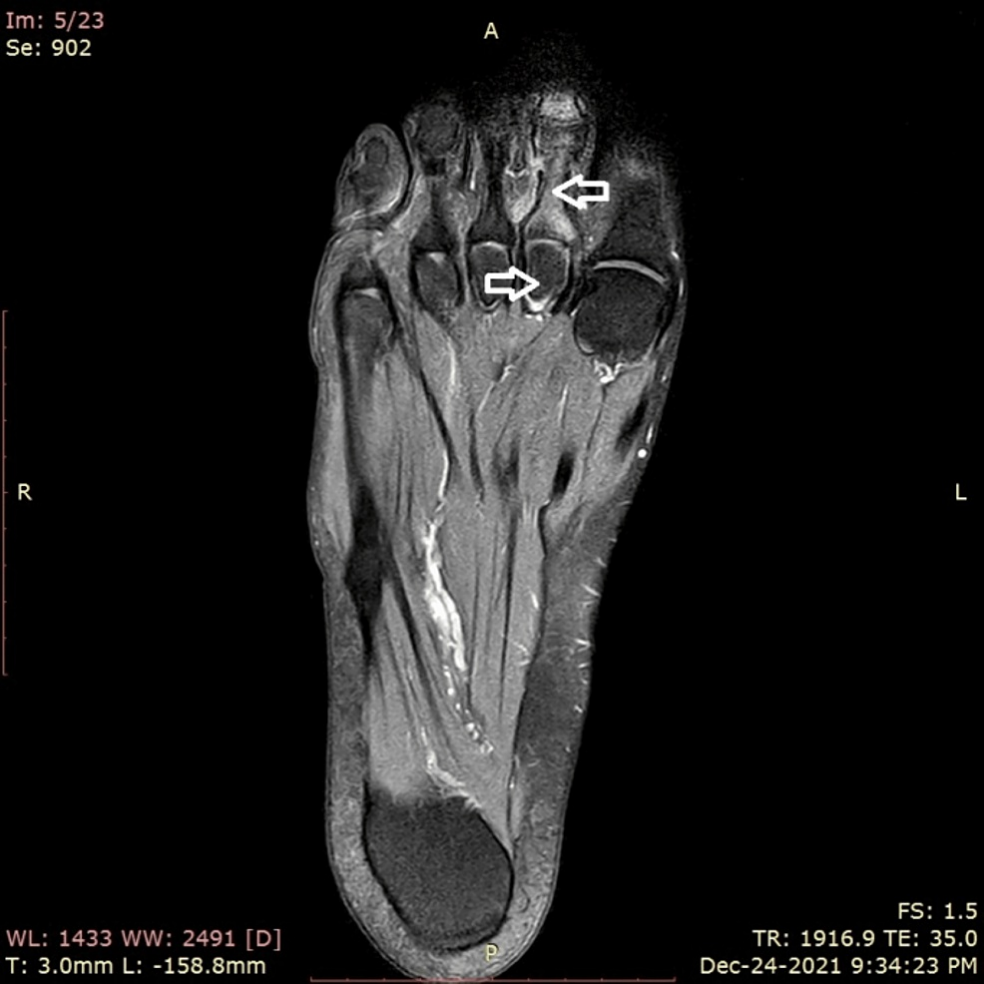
Improvement of the inflammation as compared to the previous MRI (white arrows)
